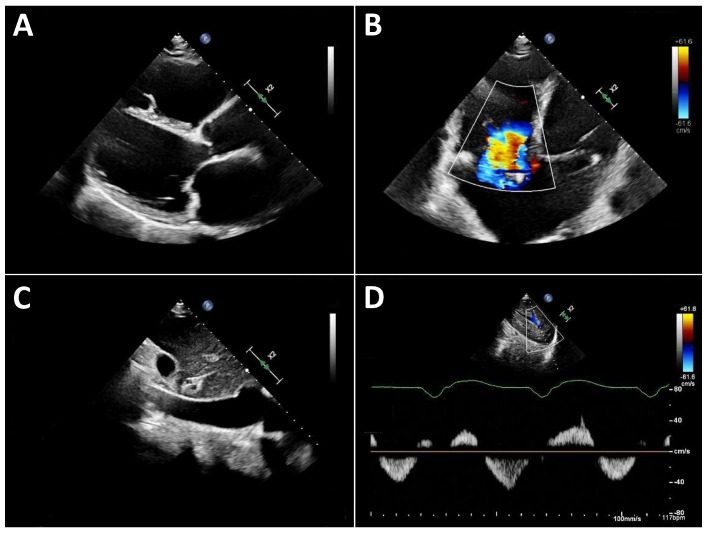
Typical echocardiographic findings of severe right heart failure in a patient with LVAD support. (A): Parasternal long-axis view showing a markedly dilated and hypokinetic proximal RVOT. (B): Apical view demonstrating pronounced right ventricular dilatation and severe tricuspid regurgitation. (C): Markedly dilated inferior vena cava with severely reduced respiratory variation. (D): Systolic flow reversal in the hepatic veins.
